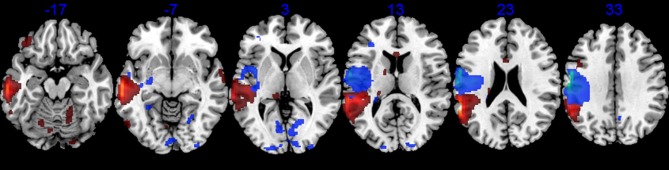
^18^FDG-PET. Axial PET images (MRIcroN) of patients with transcortical motor aphasia (blue) and conduction aphasia (red) showing significant reductions of metabolic activity in the left perisylvian areas and interconnected areas (see Table 4). Note that although the structural lesion in RTP was more superficial than the one in JGG (see Figure 2), decreased metabolic activity extended deeply to affect white matter in both cases. The left hemisphere is represented on the left side of the images.
